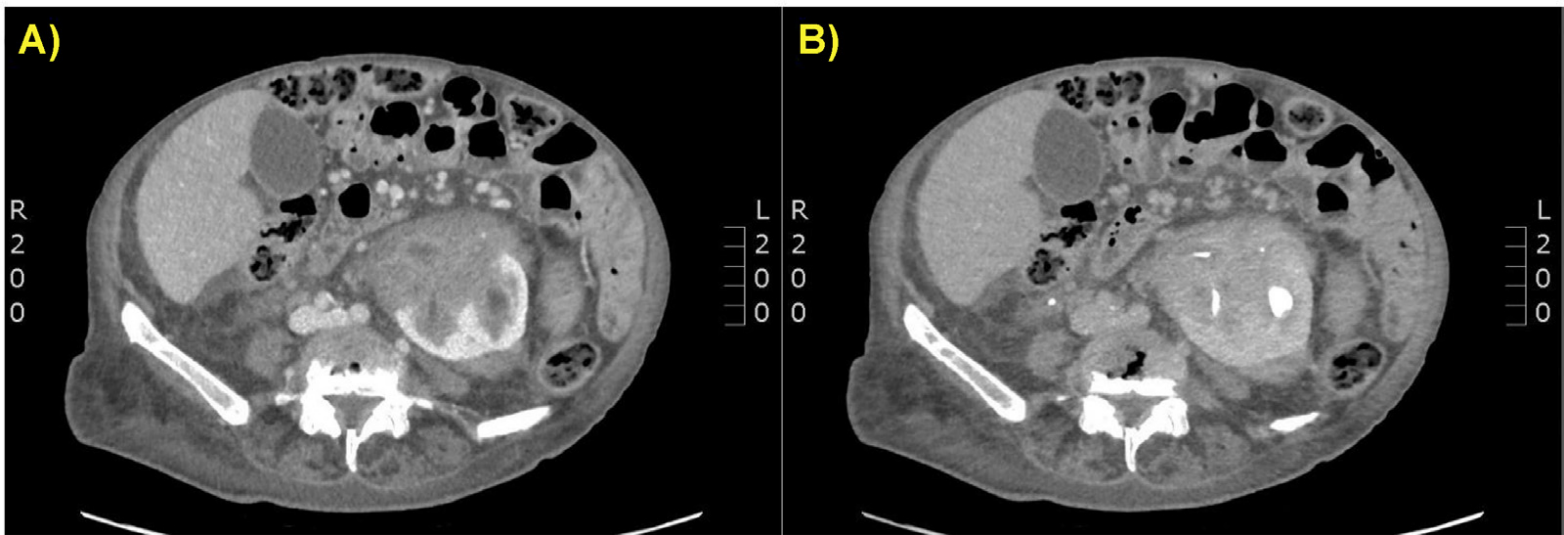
(A and B) Contrast-enhanced CT scan on axial planes. Venous (A) and delayed (B) phases showed altered morphology of the left kidney, largely occupied by an inhomogeneous hilar, and parenchymal renal area associated with a peri-renal and para-renal fluid effusion.
